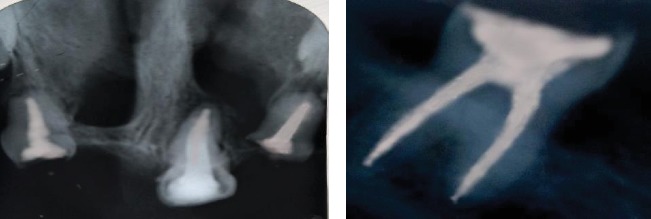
Postoperative radiograph showing root canal treated teeth with respect to teeth 11,21,22 and 46.
